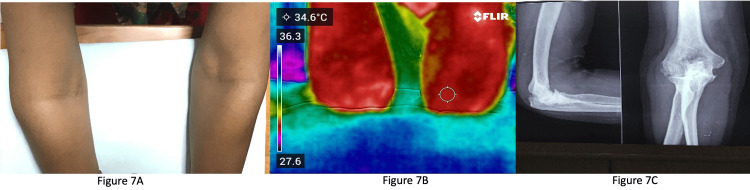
Images of a patient with a swollen right elbow 7A: Clinical image of a patient with a swollen right elbow; 7B: Infrared thermography of the right elbow joint depicting increased temperature; 7C: X-ray of the elbow joint showing reduced joint space with secondary osteoarthritis changes.
